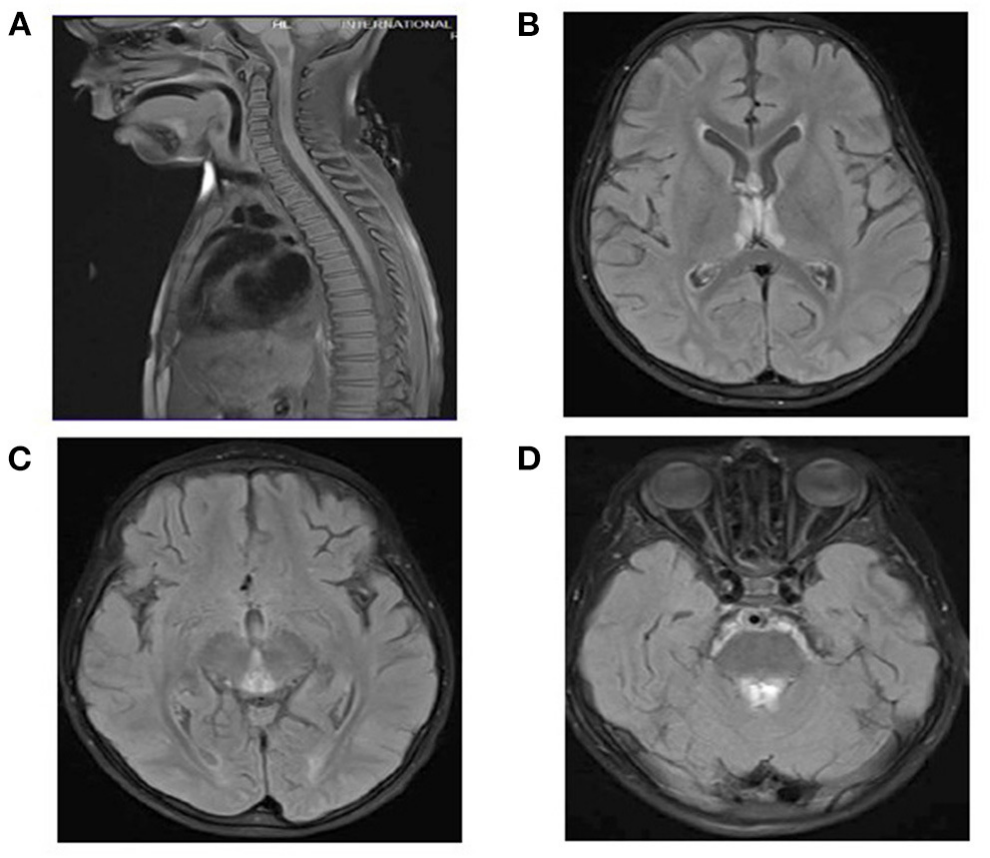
(A) Spinal MRI: Normal. (B–D) Brain MRI and FLAIR images. T2 signal hyperintensities seen on the medial thalami, dorsal aspect of the midbrain, and periaqueductal area of the midbrain.
